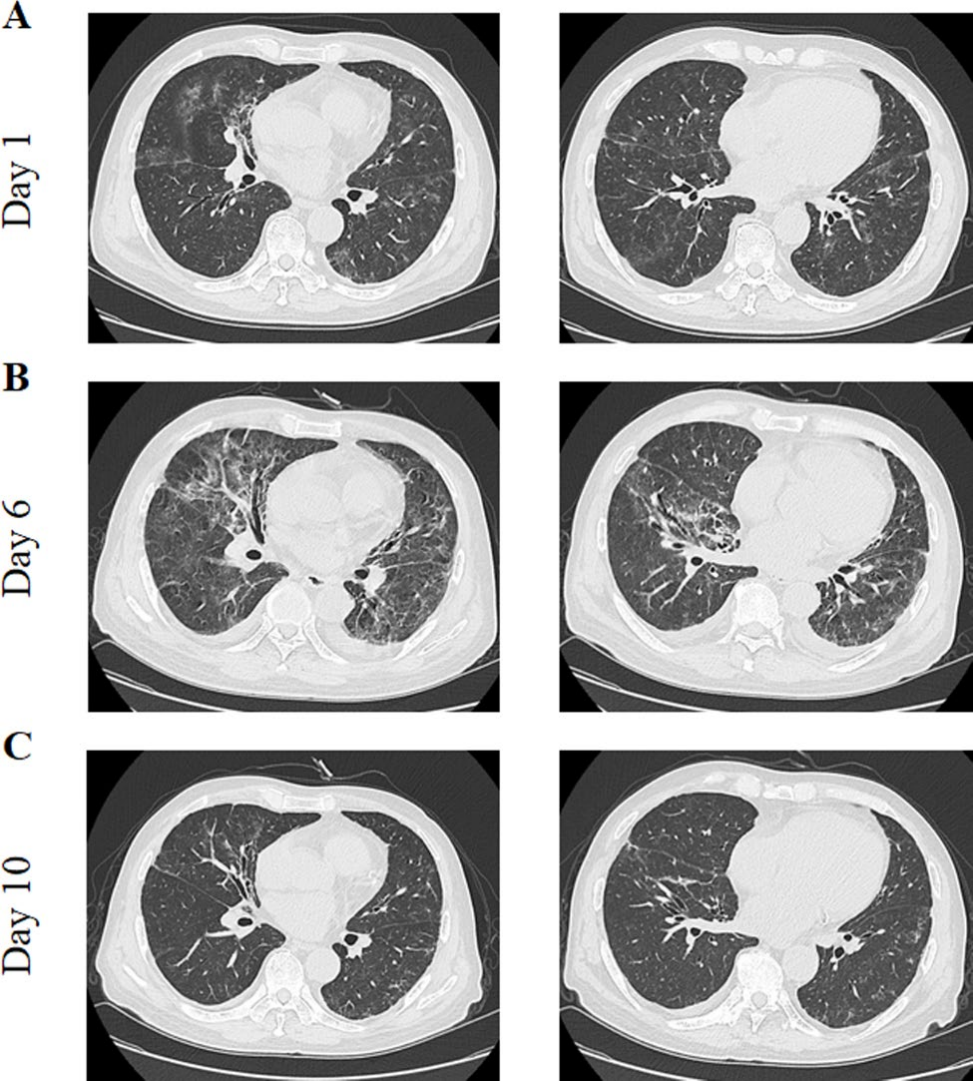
Case 1-Computed tomography images at different hospital days
